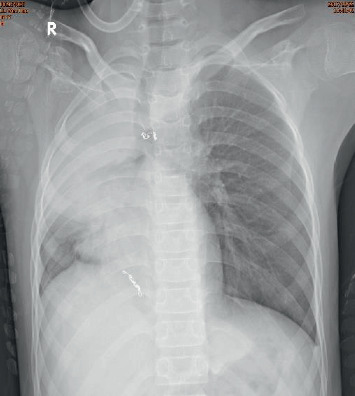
Chest X-ray documented atelectasis in the right lung.
